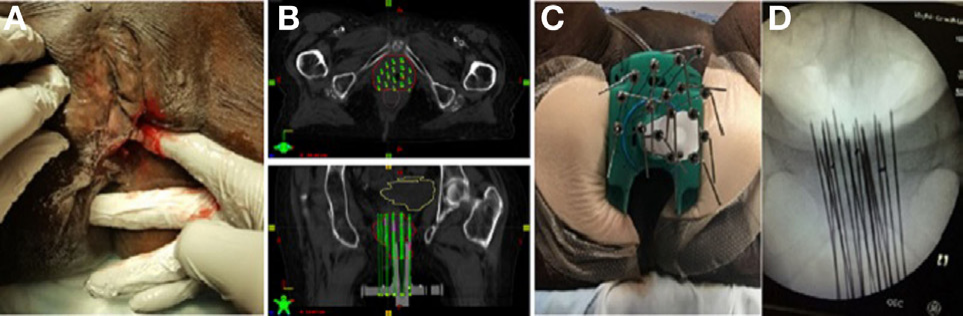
(A) Pretreatment image of tumor in right vulva. (B) Preplanning computed tomography (CT) scan for patient B with mock-up of needle placement. (C) Treatment setup for patient B with interstitial needles and modified Syed template to allow transrectal ultrasound. (D) Interstitial needle insertion under fluoroscopic guidance.
